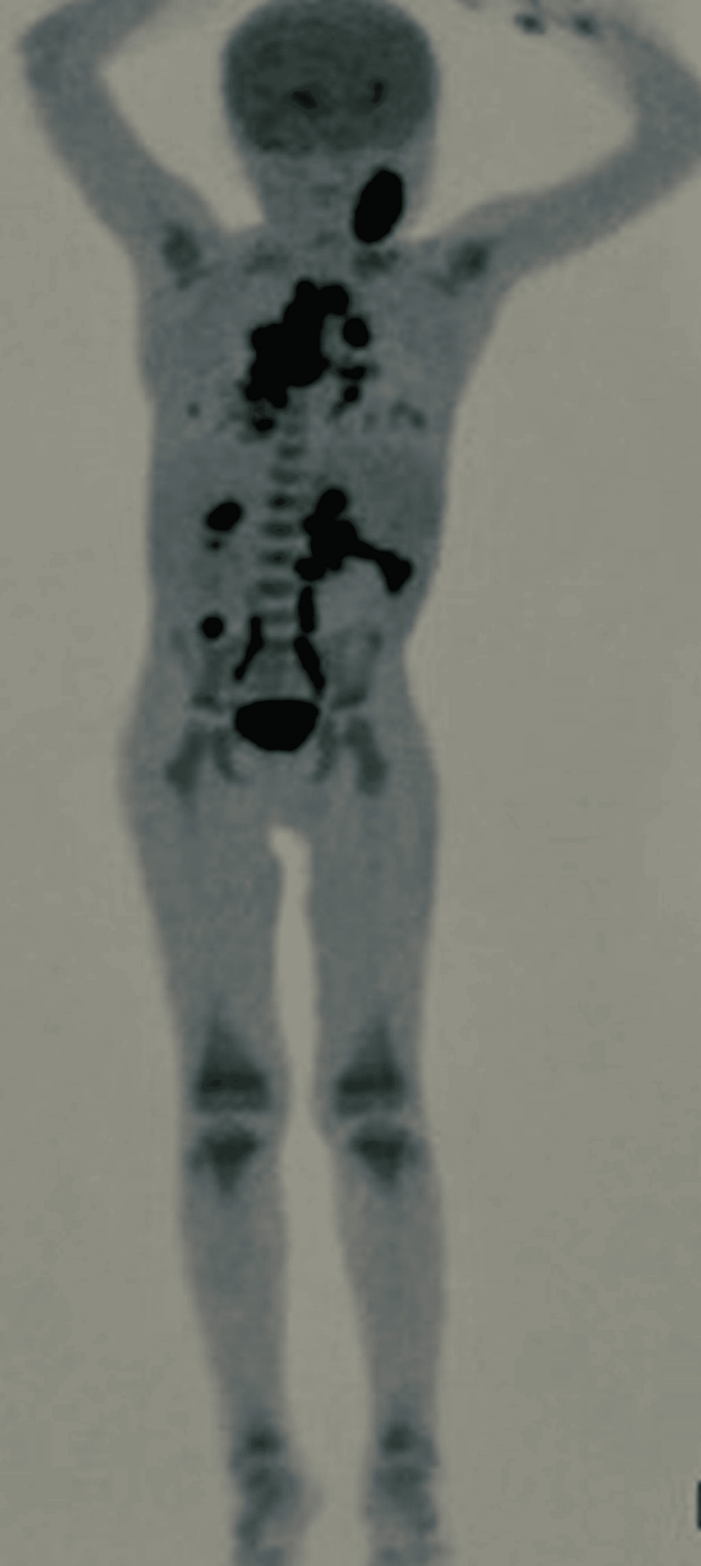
Whole body PET-CT showing conglomerate left cervical nodes, mediastinal and para-aortic lymphadenopathy
